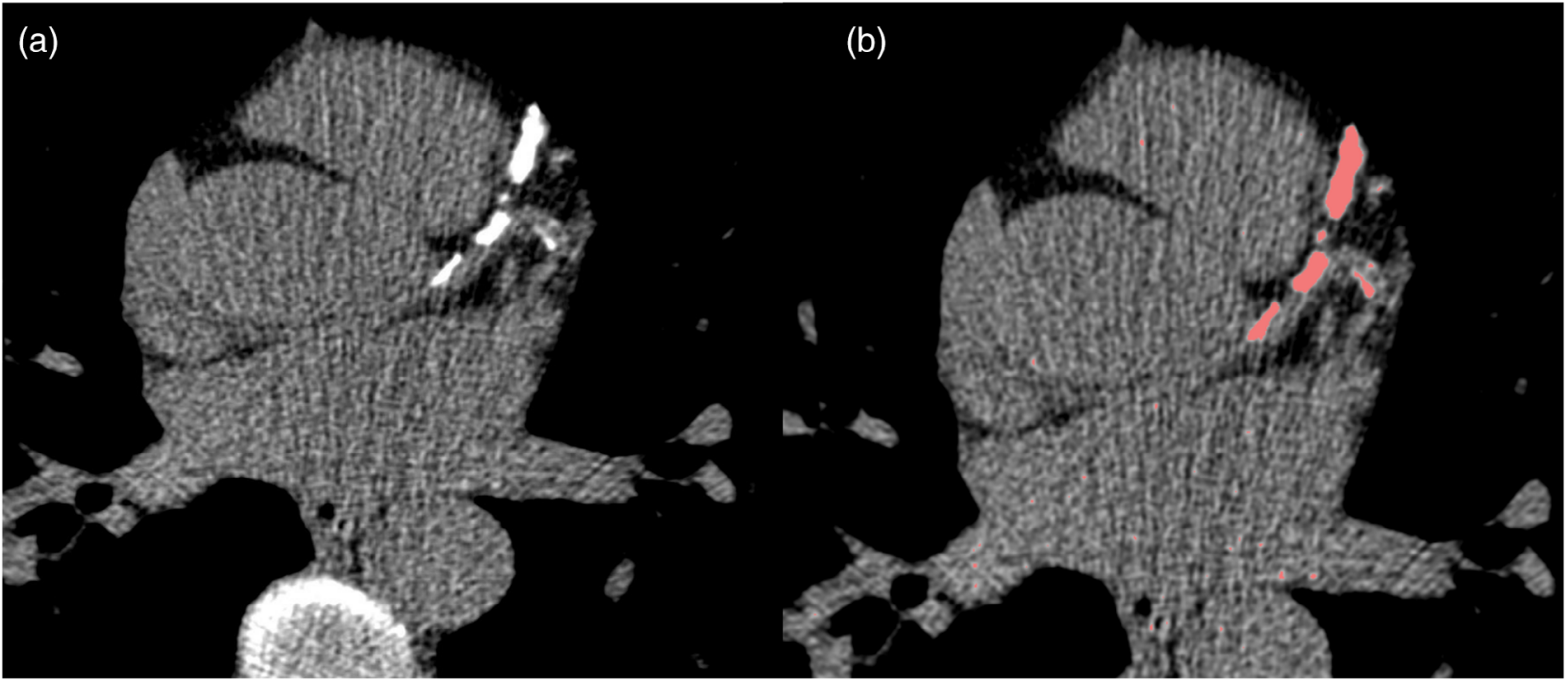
Example of an automatic measurement of CACS. Here an unenhanced CT scan is reported (a) along with the automatic segmentation of the coronary artery calcifications (b).
